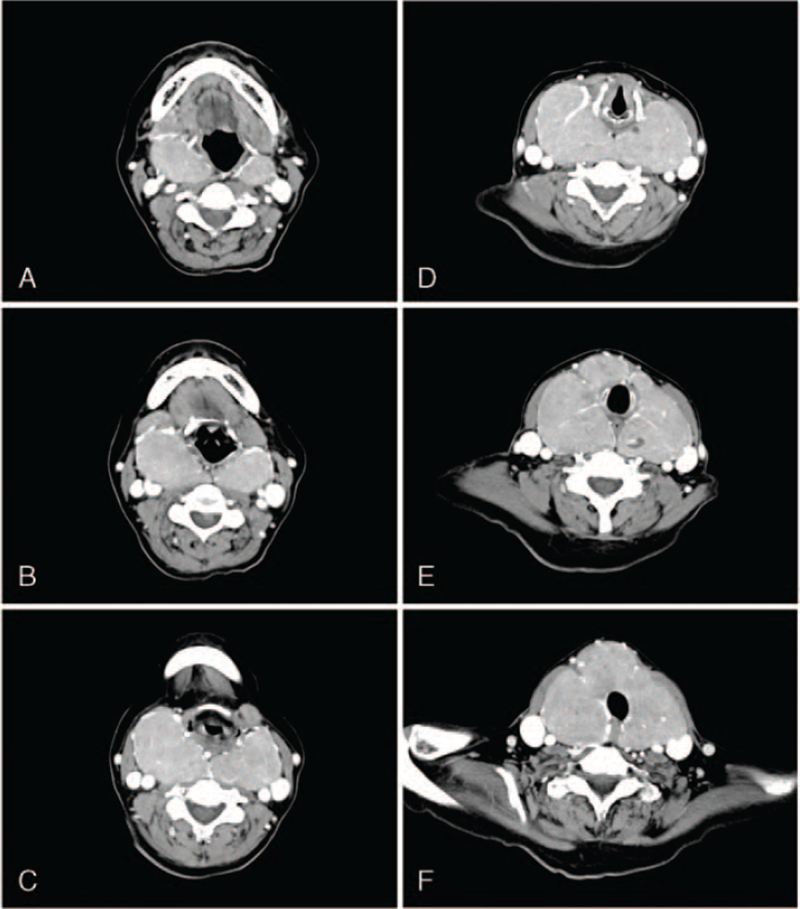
The huge goiter with bilateral retropharyngeal involvement and the trachea that was deviated leftward slightly without narrowing are seen in a series of axial computed tomography scans of the neck, showing the region from the upper structures (A) to the lower structures (F). The narrowest part of the patient airway was measured as 6.85 mm of the anteroposterior diameter and 13.45 mm of the transverse diameter in (C).
